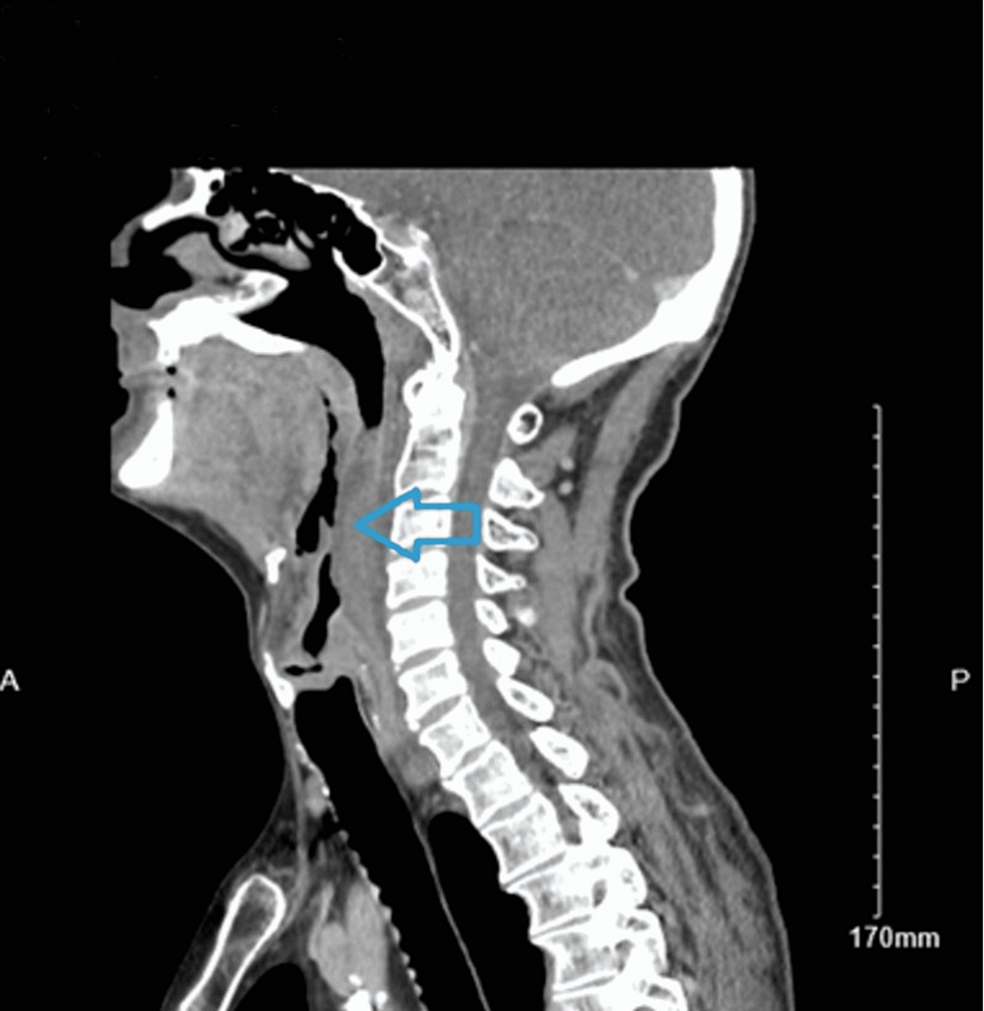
CT soft tissue neck with contrast revealing extensive asymmetric left retropharyngeal edema extending from the level of the oropharynx to the level of the larynx. There is a small 3 mm phlegmon and a marked narrowing of the hypopharyngeal airway (blue arrow). CT: computed tomography.
